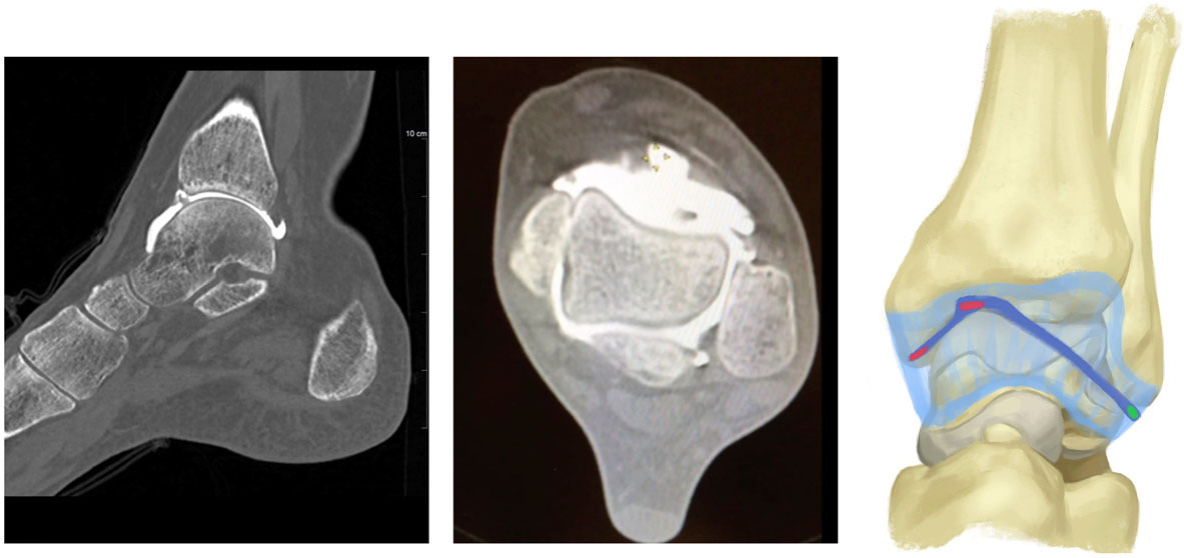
Sagittal and axial CTA views, and the shape of the fibrous band in patient 1
